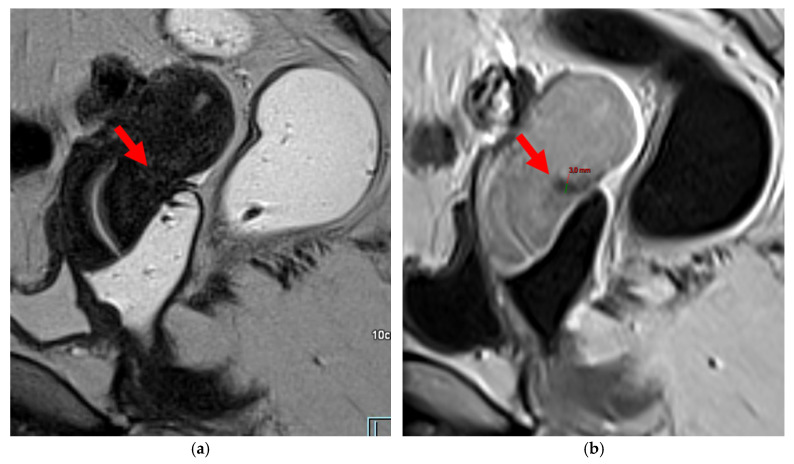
Pelvic MRI scan of a symptomatic patient with a retroflexed uterus. (a) Sagittal T2WI shows a uterus in a retroverted/retroflexed position, resulting in an unassessable torus uterinus area (*arrow*). (b) Sagittal contrast-enhanced T1WI without fat suppression highlights the thickened torus and the origins of the USLs, making them visible and measurable (*arrow*) with a thickness >2 mm.
